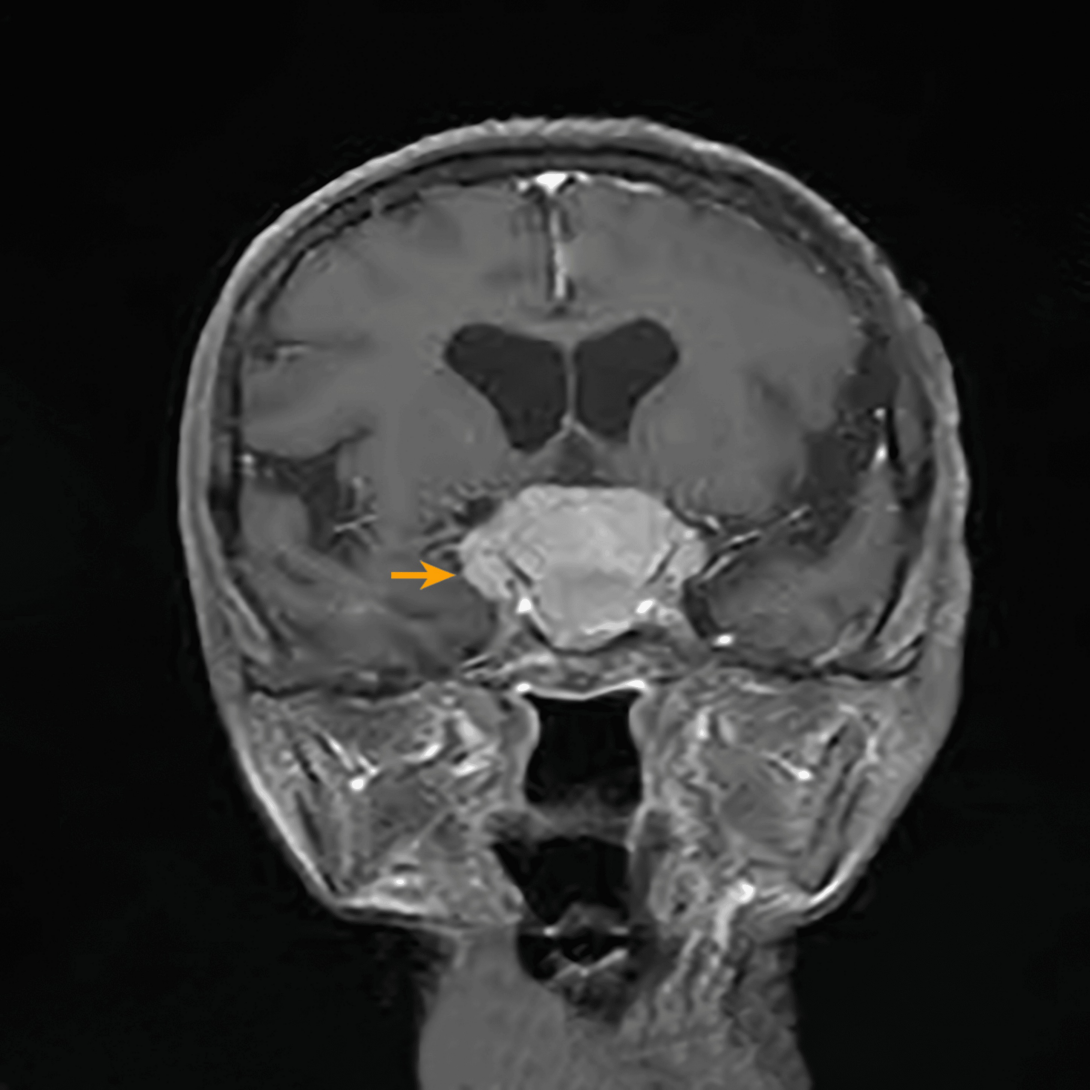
MRI gadolinium-enhanced T1-weighted coronal image, showing the tumor (orange arrow) extending anteriorly into the bilateral optic canal and planum sphenoidale region MRI: magnetic resonance imaging
